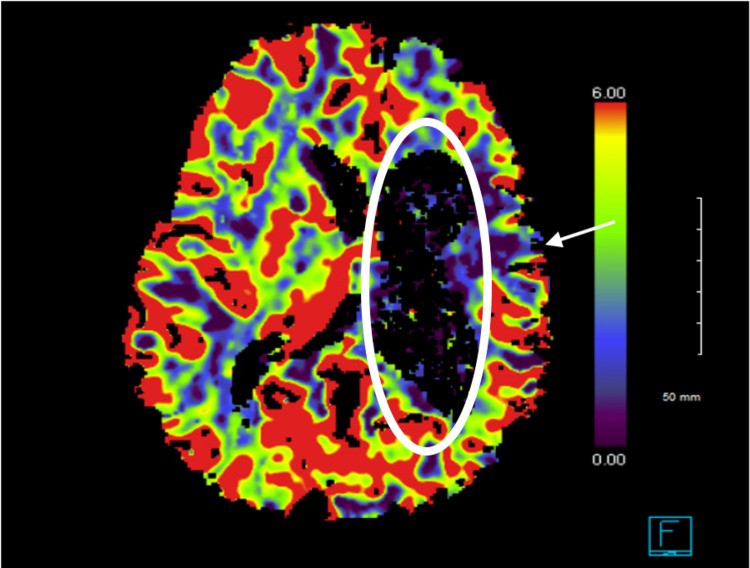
Computed tomography cerebral perfusion imaging with intravenous contrast White arrow is pointing to areas of decreased blood flow and volume identified within the left posterior temporal and parietal cortical subcortical region, and the white oval is encircling the dilated left lateral ventricle.
